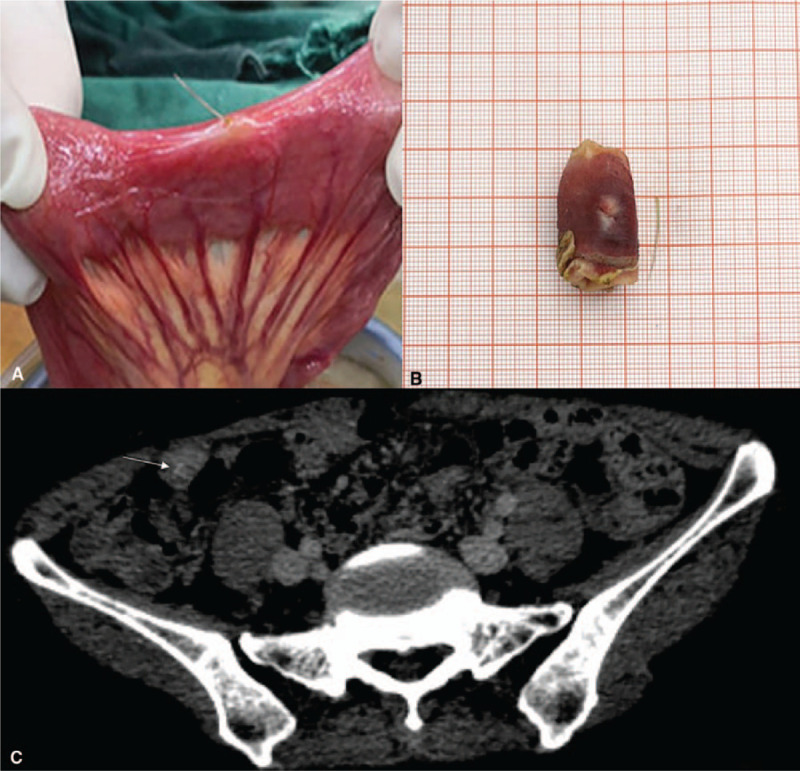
A. Operative findings showed the fish bone lodged at the end of the ileum causing perforation and coated with a small amount of fibrinous exudates. There was no definite obstruction or stricture. B. The distal ileum was removed along with the 1.7-cm-long fish bone. C. Re-evaluation with contrast-enhanced abdominal CT showed a suspected radiopaque linear shadow at the end of the ileum lodged into the thickened intestinal wall. There was no evidence of localized inflammation or fluid collection. (white arrow).
